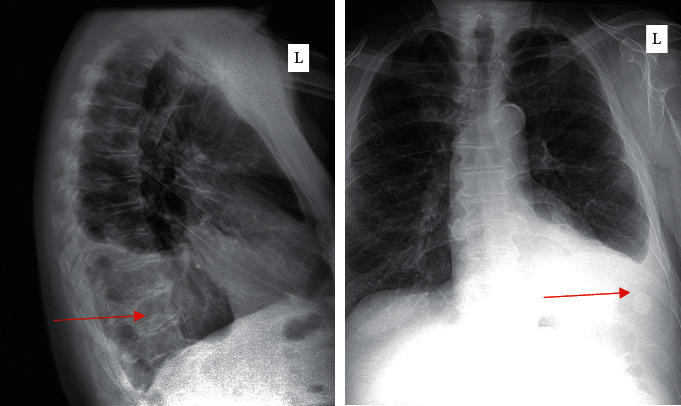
Lateral and posterior-anterior chest X-ray showing left-sided pleural effusion (arrows) during symptom relapse.
